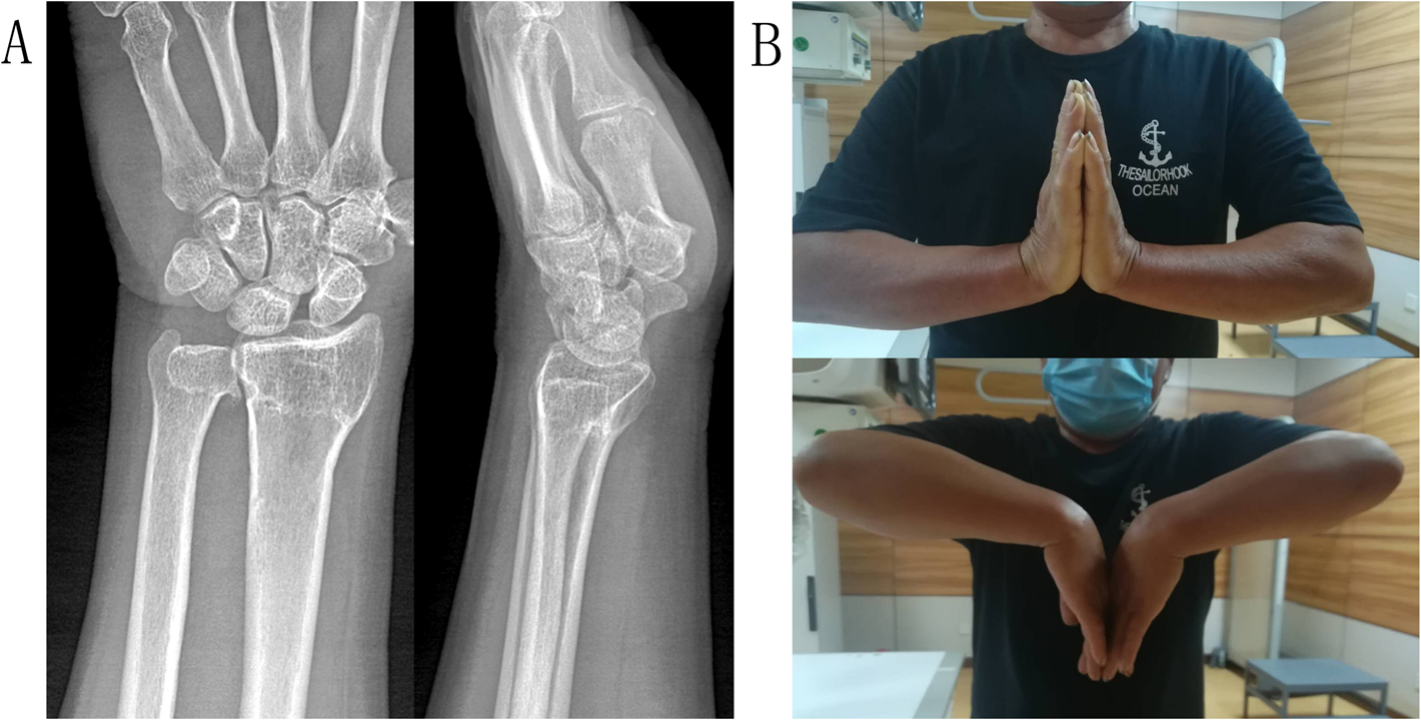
The frontal and lateral X-ray films (A) showed evidence of the excellent fracture healing of 26 months postoperative. At the last follow-up, the affected wrist achieved an excellent function (B). The VAS score was 0, the modified Mayo wrist score was 95, and the *Quick*DASH score was 2.27
